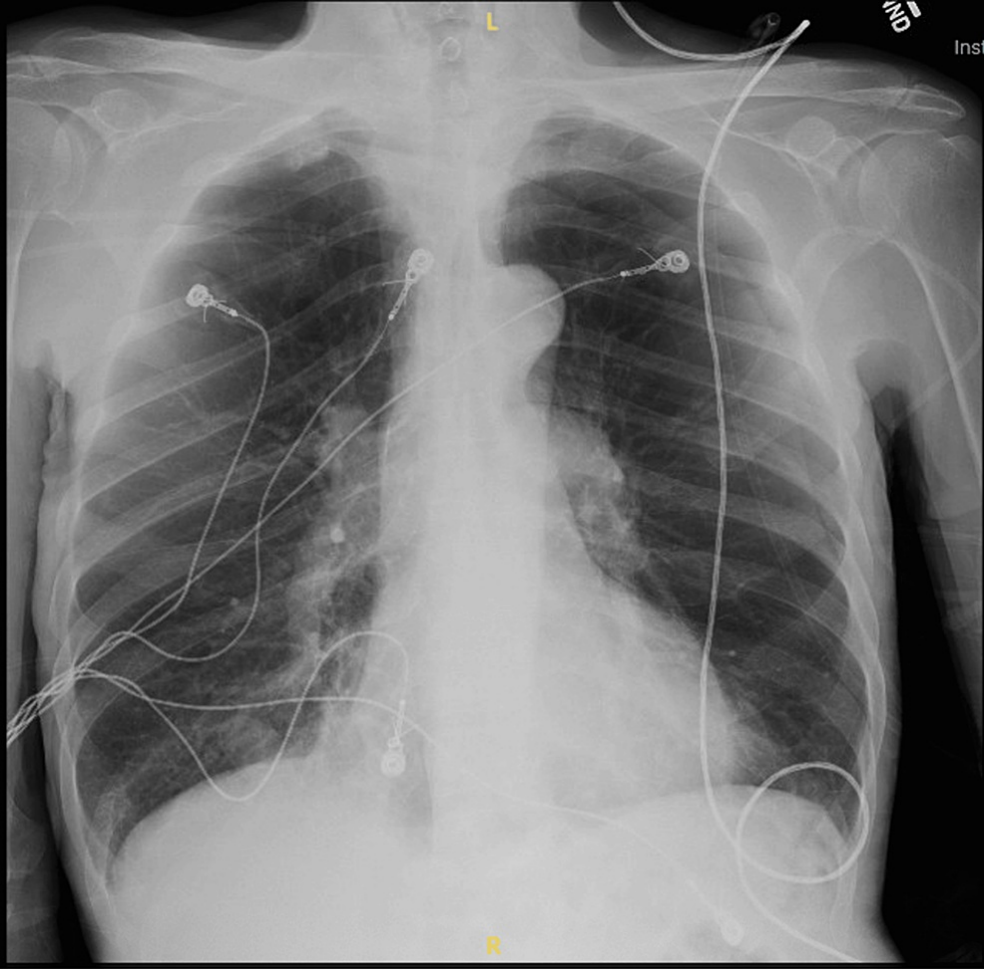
Chest radiography with no acute process identified.
